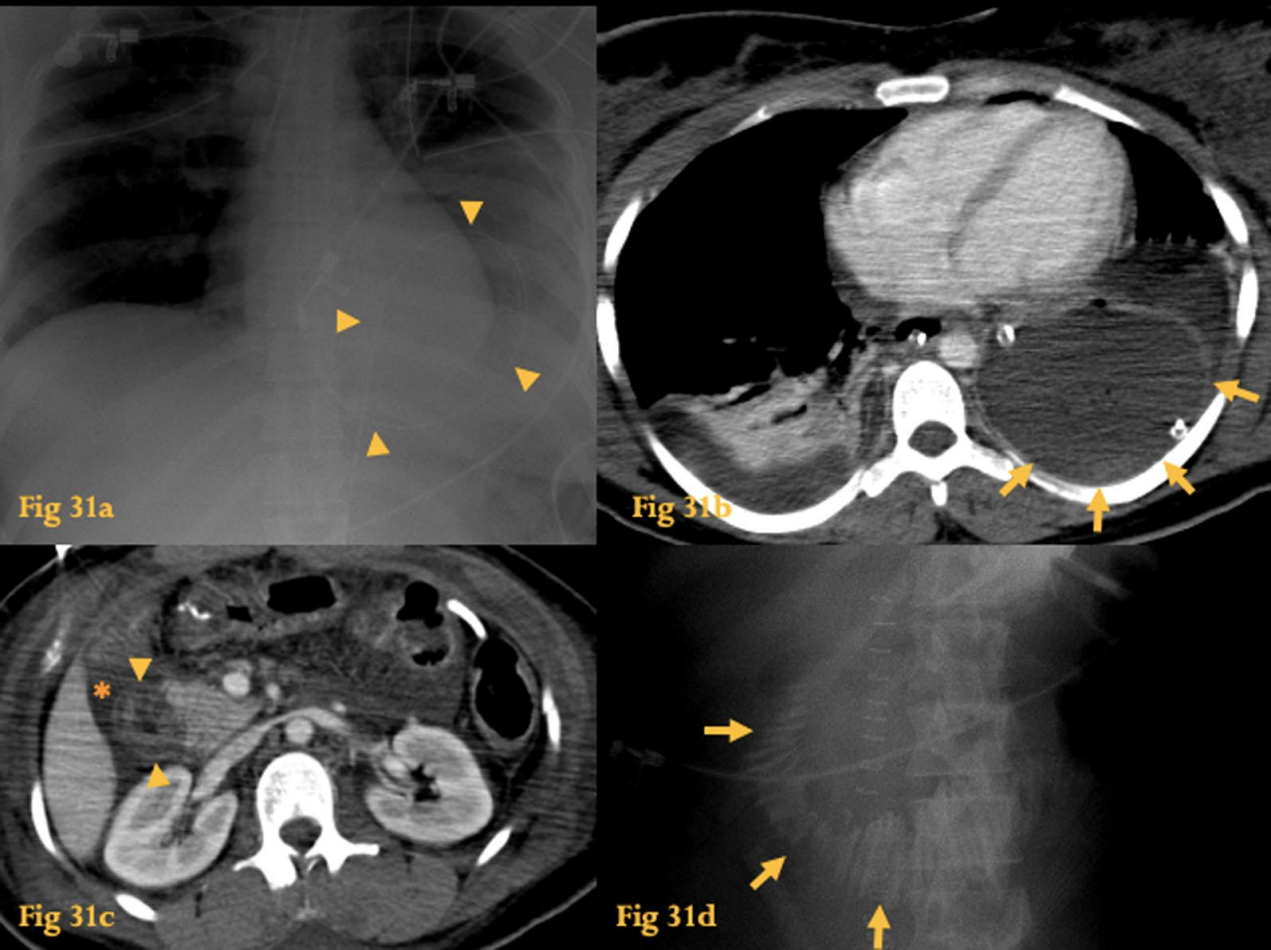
Trauma. Chest radiograph (a) of a car accident victim shows a gastric tube with a thoracic loop (arrowheads). Contrast-enhanced CT of the same patient (b) confirms traumatic diaphragmatic hernia; note the absence of interposition of lungs between the stomach and the chest wall—the so-called “dependent viscera sign” (arrows). Abdominal CT (c) shows that D2 is surrounded by fluid (asterisk) and its wall presents the target sign (arrowheads). The upper GI study (d) of the same patient revealed uniform, regular, fold thickening at D2 and D3, similar to a stack of coins (arrows), representing intramural edema/hematoma
